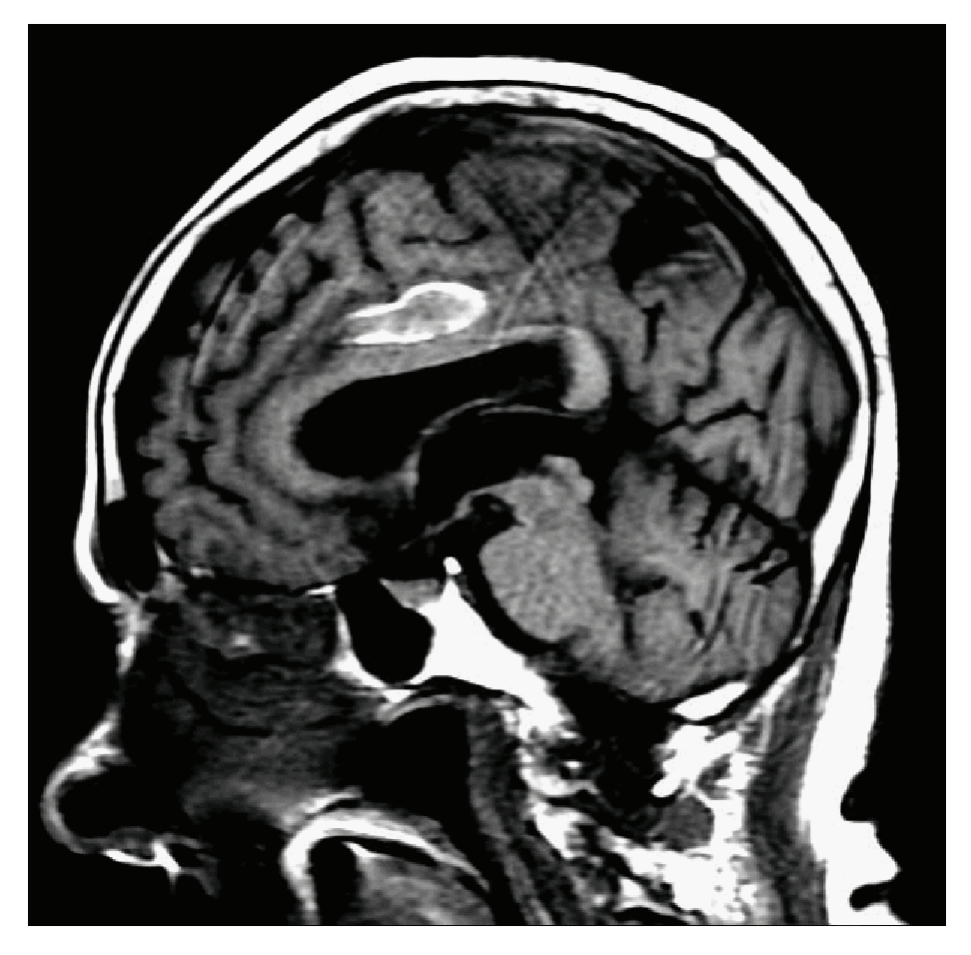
Sagittal MRI T2-FLAIR showing hyperintensity in the parasagittal region compressing corpus callosum and extending into the left parasagittal region.
